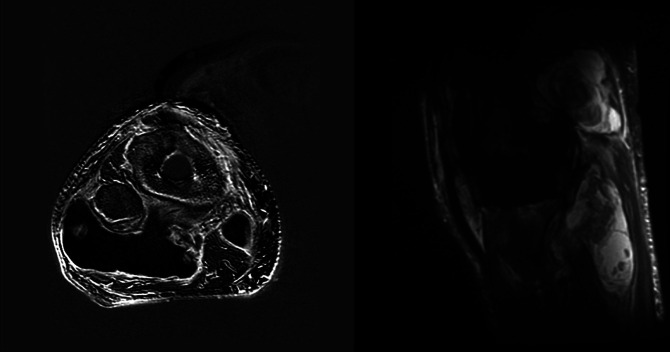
Radiograph showing preoperative metal suppression MRI (axial left and sagittal right) demonstrating abnormal signal around the total knee arthroplasty implants with associated large fluid collections containing heterogenous debris.
